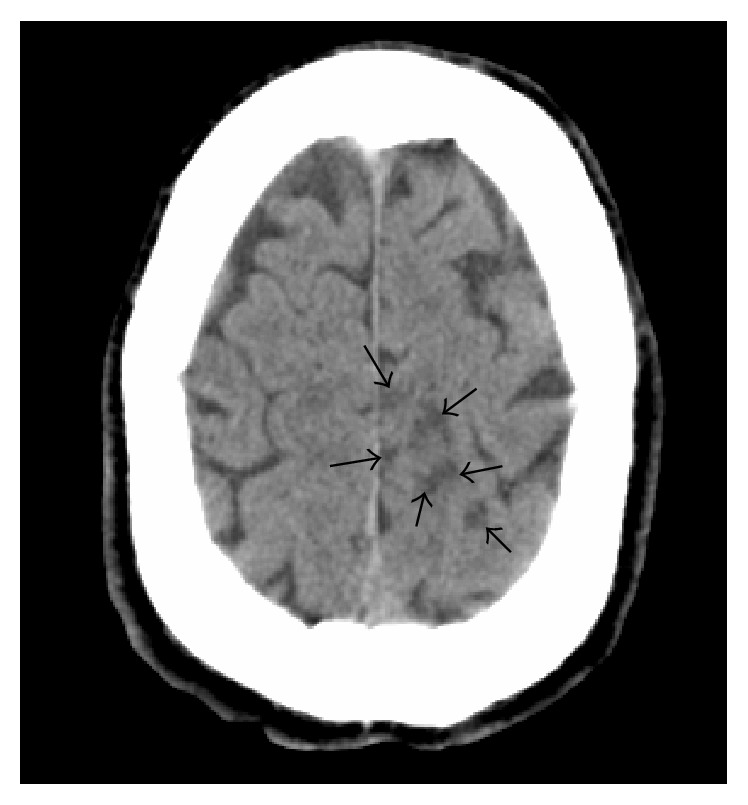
Brain CT showing new multiple, diffuse hypodense lesions near the vertex in the left hemisphere (arrows).
